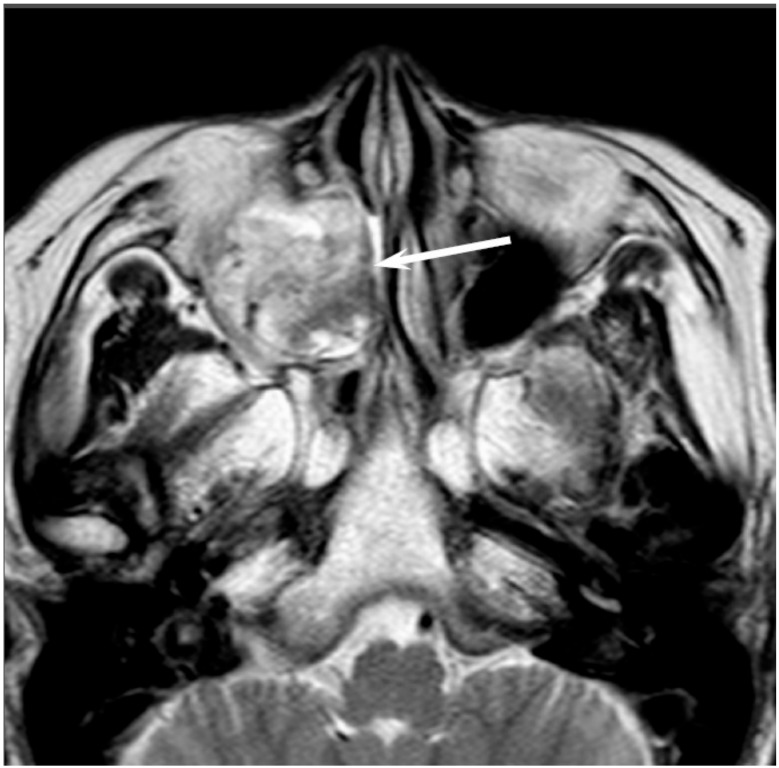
Non-intestinal type adenocarcinomas of the right maxillary sinus. T2-weighted image showing a heterogeneously hyperintense lesion (arrow).
